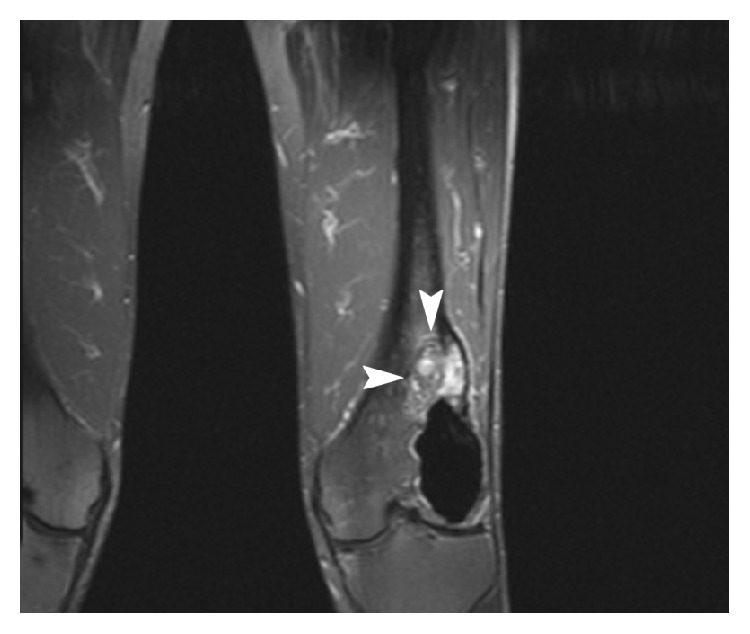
Coronal T1 postcontrast CT (November 2013) demonstrated a heterogeneously enhancing soft tissue mass (arrows) within the lateral aspect of the distal femoral condyle, proximal to the hypointense focus of PMMA.
